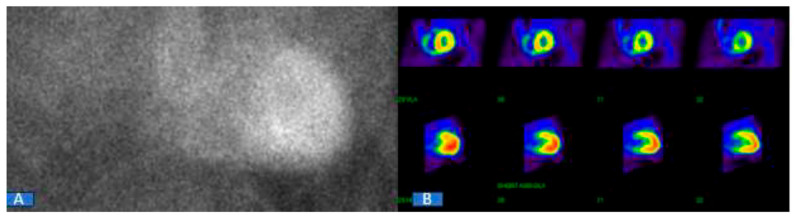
Positive 99mTc-labeled nuclear scintigraphy. (A) Visual grade 3 on planar image; (B) diffuse myocardial tracer uptake on single-photon emission computed tomography.
